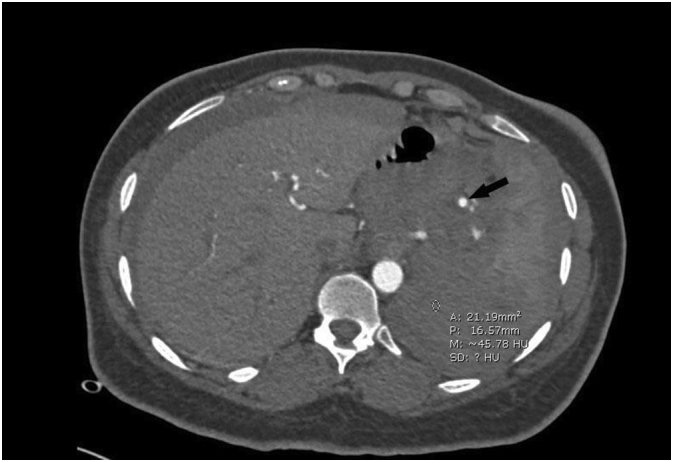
Shows Abdominal CT angiogram arterial phase, the arrow points at the site of the aneurysm.
